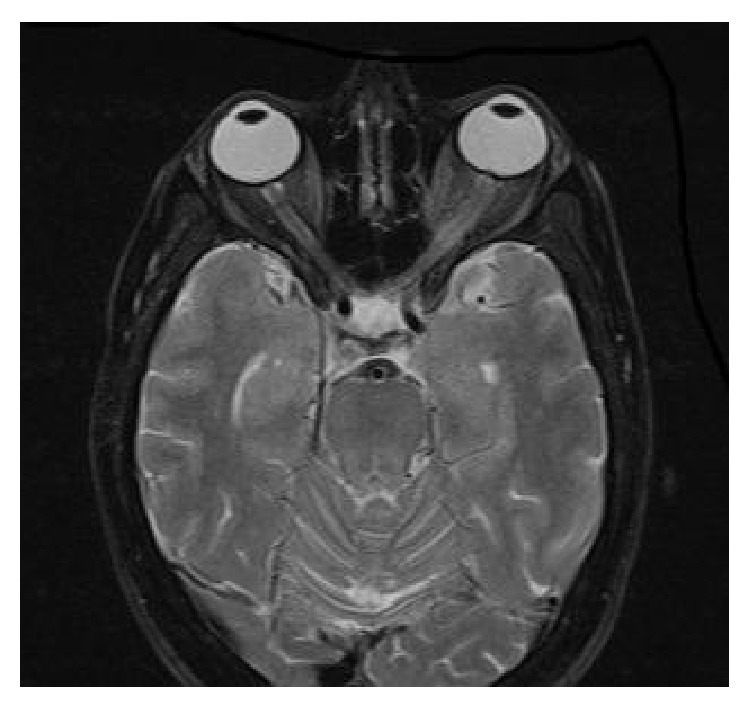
Cranial magnetic resonance imaging of neuropathy in the left optic nerve.
